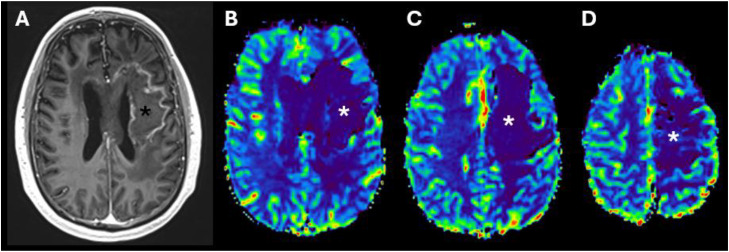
Brain magnetic resonance imaging (MRI) performed while the patient was still receiving antiangiogenic therapy, including axial post-gadolinium T1-weighted MPRAGE volumetric sequence (A) and relative cerebral blood volume (rCBV) T2*-weighted perfusion maps (B–D). The lesion corresponding to treatment-related necrosis (asterisks) demonstrates peripheral contrast enhancement and marked hypoperfusion (low rCBV). Careful evaluation of the perfusion pattern in the left frontal lobe also reveals mild hypoperfusion.
